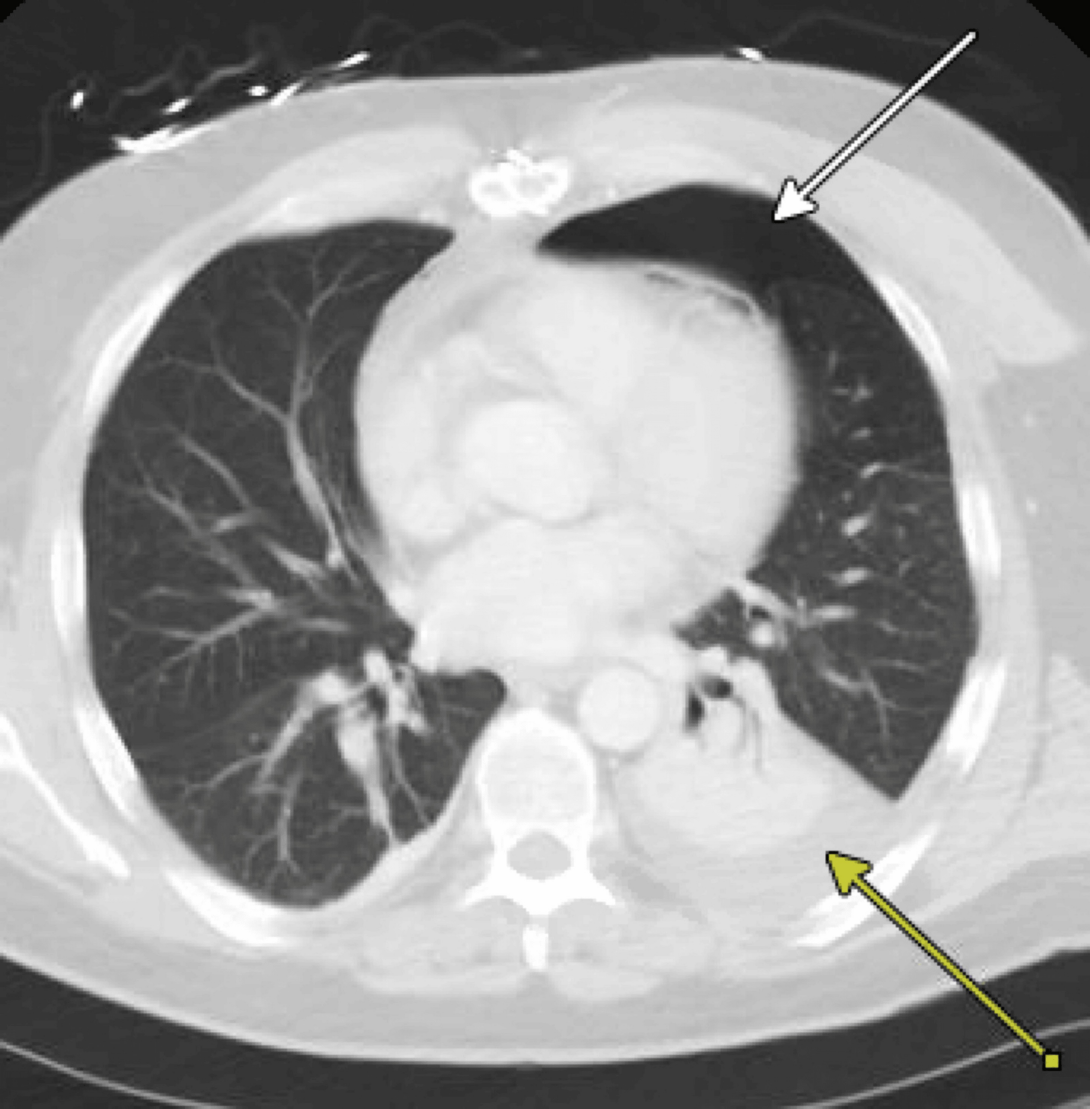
Case 2: CT scan of the chest post-discharge Left-sided hydropneumothorax with labels on the pneumothorax (white arrow) and hemothorax (yellow arrow)
